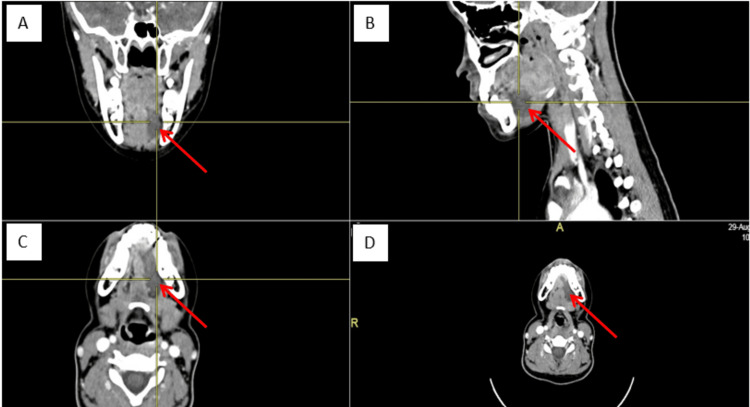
A-D: Coronal, sagittal, and axial view of CT scan revealed a hypodense lesion measuring 4x4 cm, confined to left sublingual space, which displaced left genioglossus medially, digastric muscle inferiorly, bounded laterally by mylohyoid muscle and abutted the base of the tongue superiorly A: Coronal view; B: Sagittal view; C-D: Axial view
